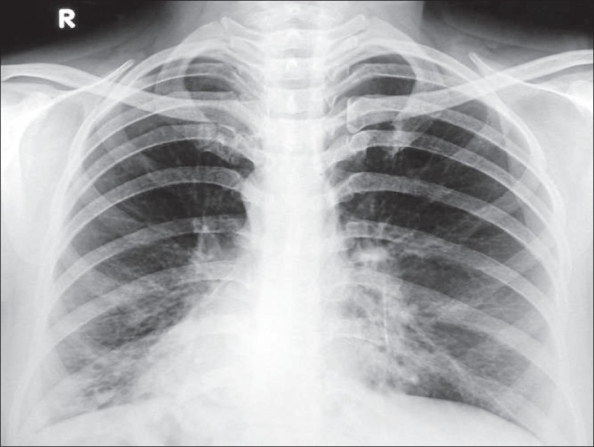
Chest radiograph PA view
